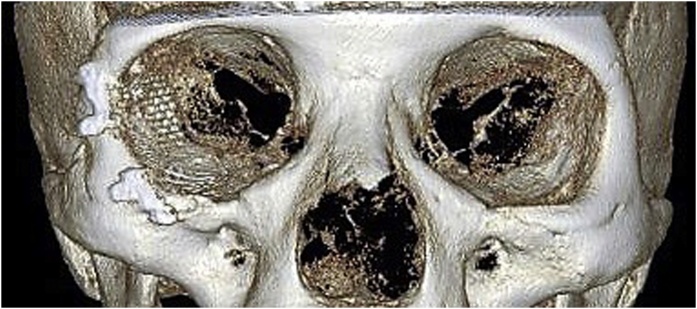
Postoperative CT showing the graft and titanium mesh probably adapted.
